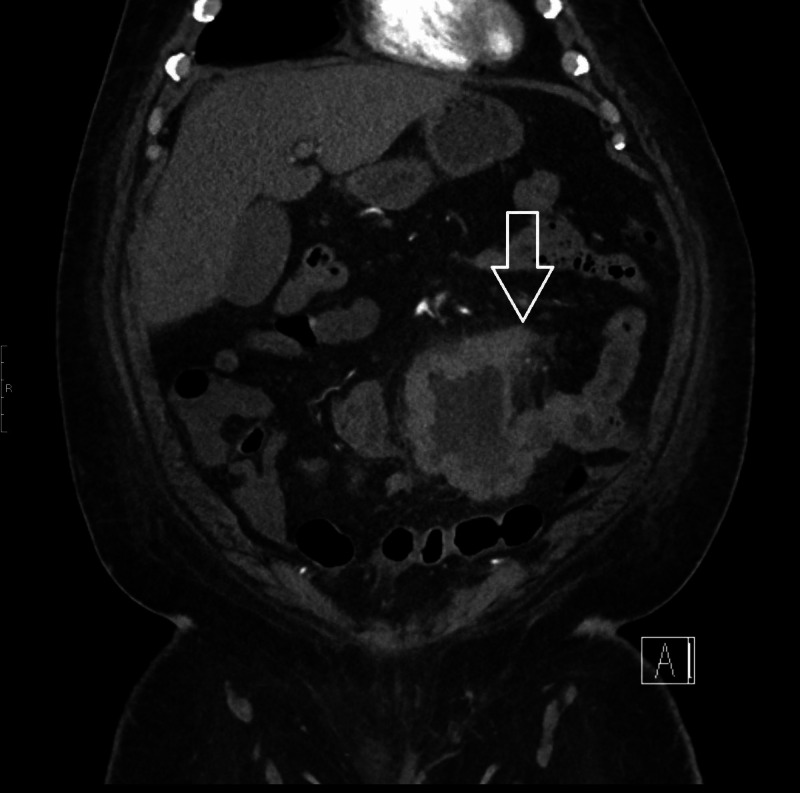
CT scan of the abdomen and pelvis: coronal view
